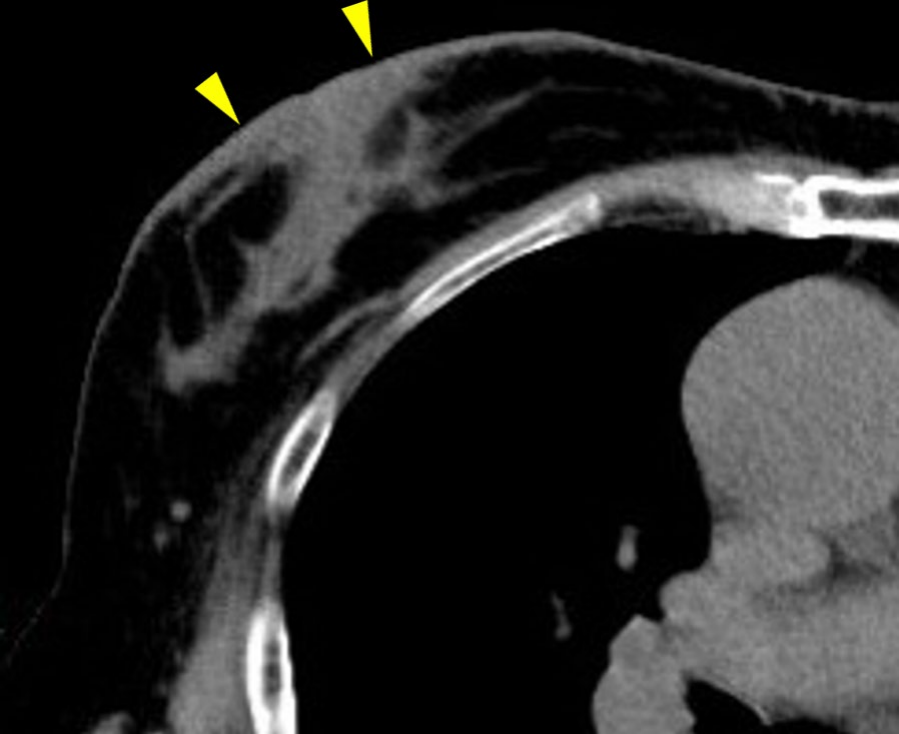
Computed tomography of the chest. Yellow arrowheads indicate skin thickening of the right breast
